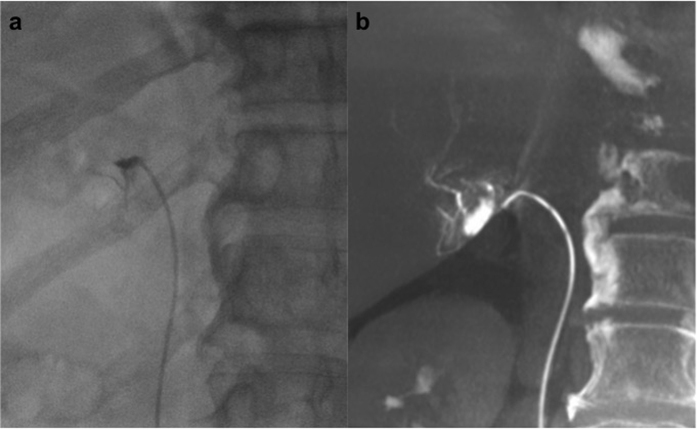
A 61-year-old man with primary aldosteronism (PA) and a right adrenal nodule. (a) The venogram pattern of the accessory hepatic vein was similar to that of the right adrenal vein. (b) Dyna computed tomography exhibited focal opacification of the liver parenchyma, with venous drainage into the accessory hepatic vein.
